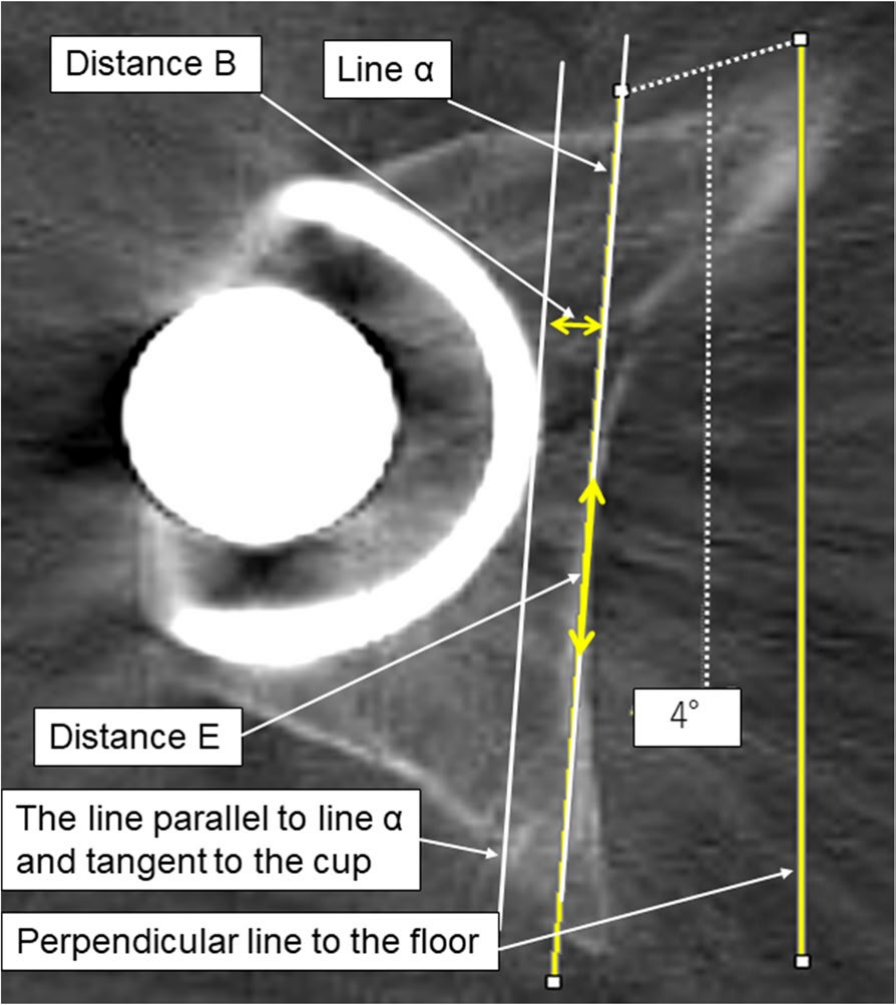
Measurement of distance B. In the axial CT image at the level of the hip joint center, the distance between the metal cup and line α tangent to the medial acetabular wall with maximum overlap was measured as shown. CT, computed tomography
